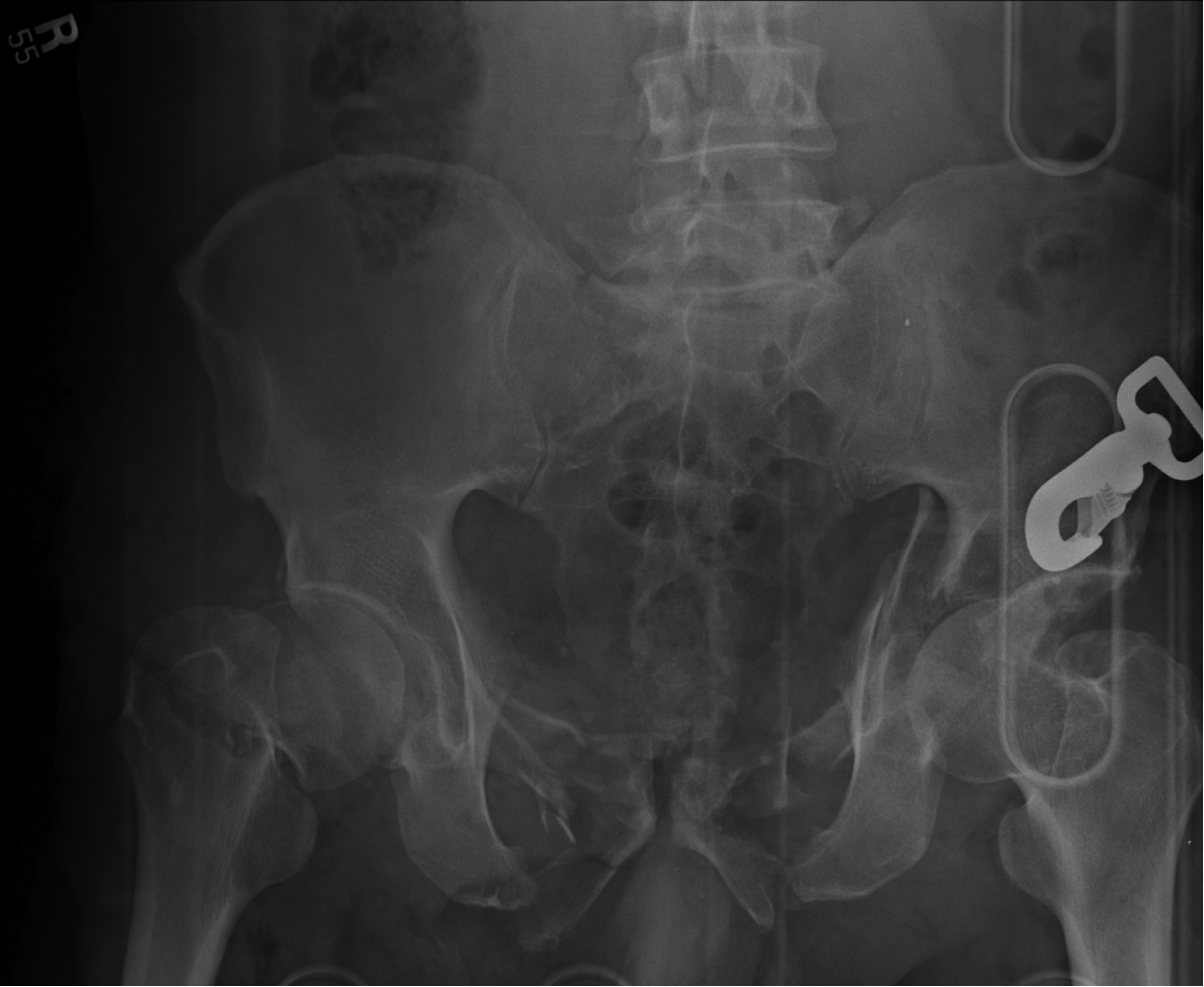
Patient # 10 - Pelvis X-Ray.
